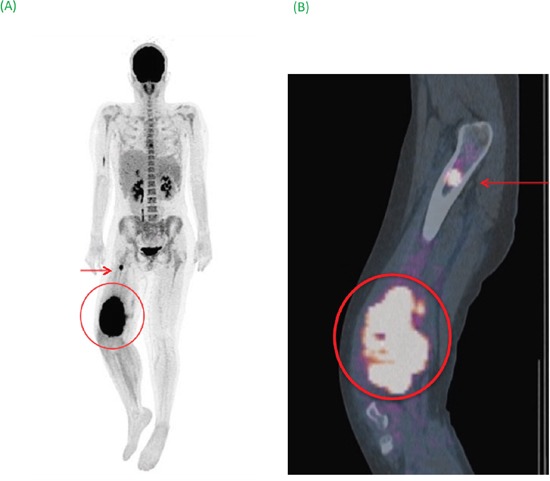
(A) Maximum image projection (MIP) showed the primary tumor surrounded associated with bone metastatic site (arrow). (B) Sagittal fusion FDG-PET/CT image specified lesions’ anatomical locations. Semi-quantitative PET parameters of the primary tumor (SUV_max_ of 29 and SUV_peak_ of 20.57) were associated with a poor prognosis (OS: 9 months).
